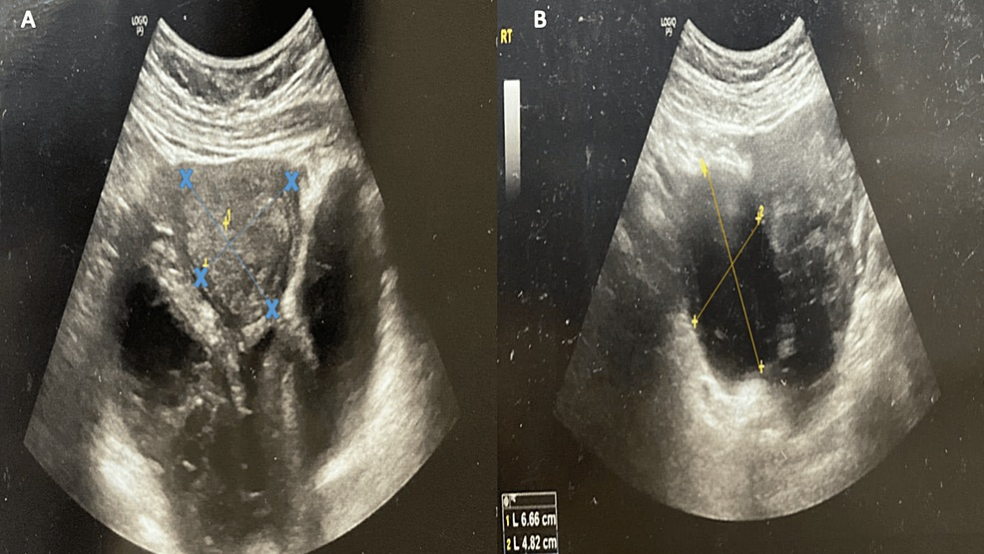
A transabdominal sonographic that shows a lesion on the left side (A), which is a complex, cystic, and multiloculated with several septations and echogenic material that appears to be retracting. It measures 5.92 x 4.74 cm and exhibits low vascularity. On the right side (B), a simple cyst is present with a thin wall. This cyst is characterized by hemorrhagic echogenicity and measures 6.6 x 4.8 cm. A minimal amount of free fluid is also detected in the pelvic area.
